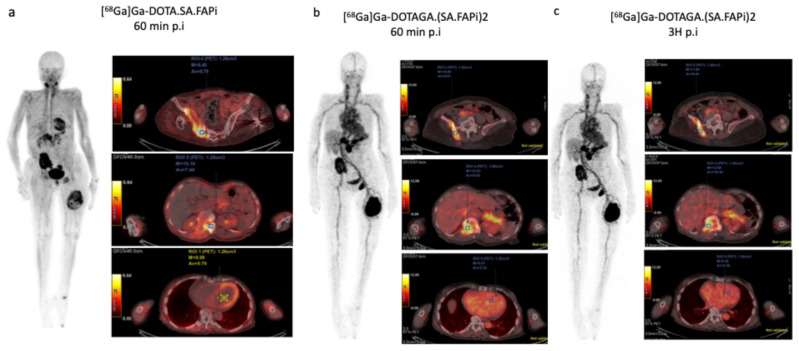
A 63-year-old woman with papillary thyroid carcinoma underwent FAPI PET/CT imaging. (a) Maximum-intensity projection (MIP) images of [^68^Ga]Ga-DOTA.SA.FAPi obtained at 60 min post-injection demonstrates physiological tracer uptake in the salivary glands, pancreas, and myocardium, along with multiple FAPI-avid skeletal lesions involving the right ilium, left ischial tuberosity, and left femur. (b,c) MIP images acquired using [^68^Ga]Ga-DOTAGA.(SA.FAPi)_2_ at 60 min and 3 h post-injection, respectively, show physiological biodistribution predominantly in the pancreas and liver, with concordant radiotracer uptake in all previously identified lesions; however, lesion conspicuity and tumor-to-background ratios are notably higher compared with [^68^Ga]Ga-DOTA.SA.FAPi. Prolonged blood-pool retention of [^68^Ga]Ga-DOTAGA.(SA.FAPi)_2_ is observed, persisting up to 3 h post-injection. Fused axial PET/CT images demonstrate higher standardized uptake values for [^68^Ga]Ga-DOTAGA.(SA.FAPi)_2_ in normal organs (b,c) and tumor lesions compared with [^68^Ga]Ga-DOTA.SA.FAPi (a) [18].
